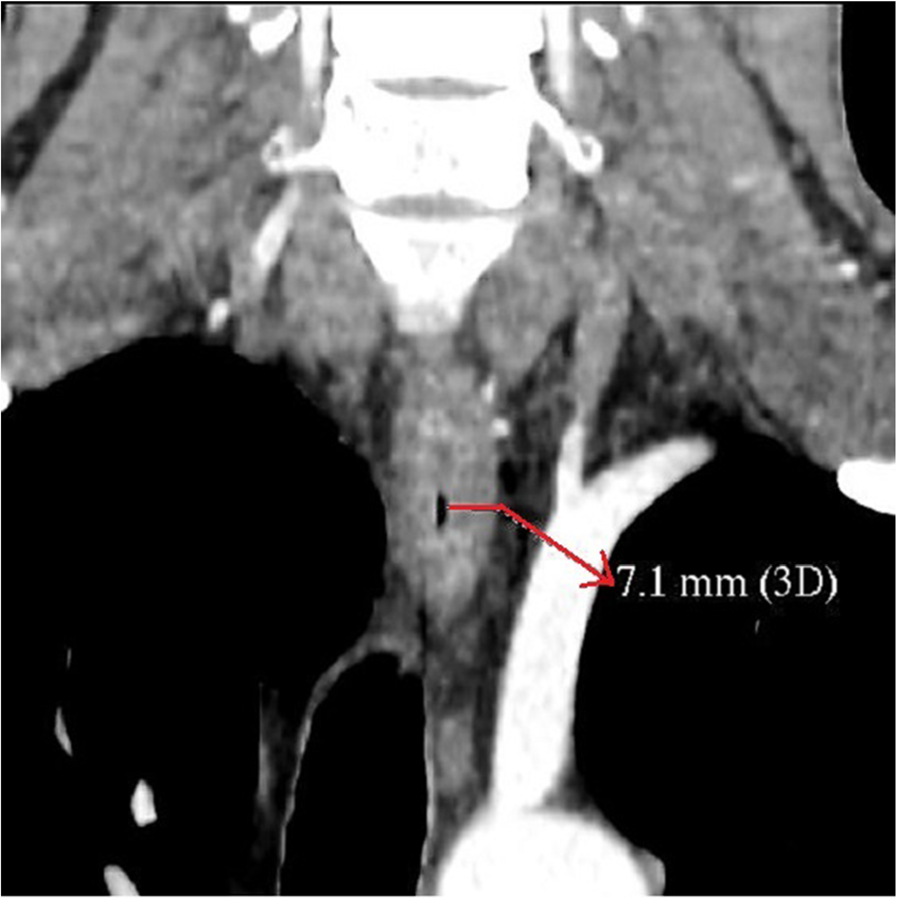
A thickening of the esophageal wall in a CT scan
